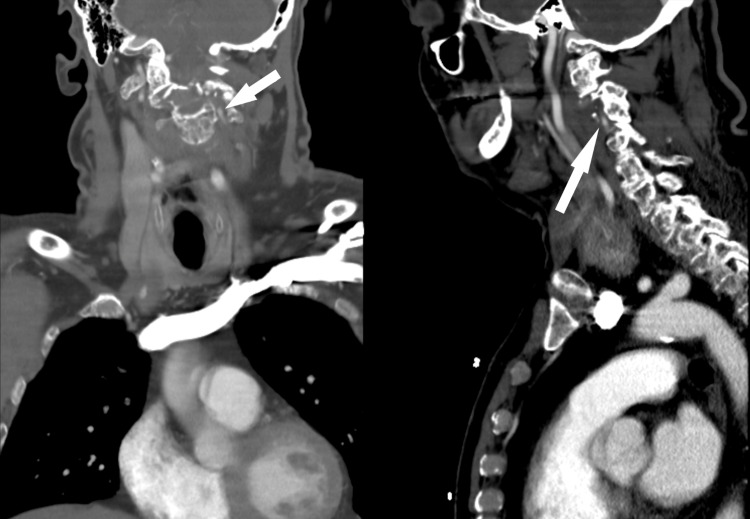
CT angiograms indicating the point of vertebral artery dissection and complete loss of contrast enhancement of the left vertebral artery at the C2 level. There is a long segment of narrowing with mural thickening of the left V3 segment indicative of a VA dissection, vessel comes to a tapering point as it is compressed by the pathological fracture of C2, and moderate focal extrinsic compression of the distal right V2 segment due to pathologic fracture of C2.
